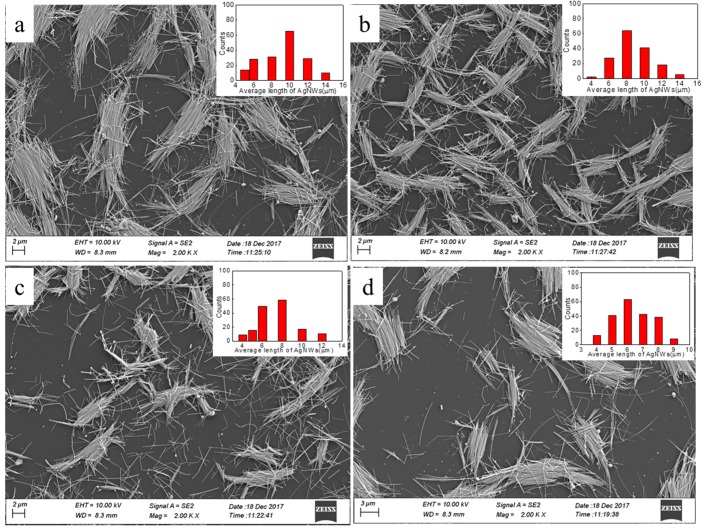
SEM images of AgNWs treated with ultrasonic power 120 W and different ultrasonic time. Ultrasonic time: (a) 1 h, (b) 2 h, (c) 4 h, (d) 5 h. The inserted images are the length distribution.
